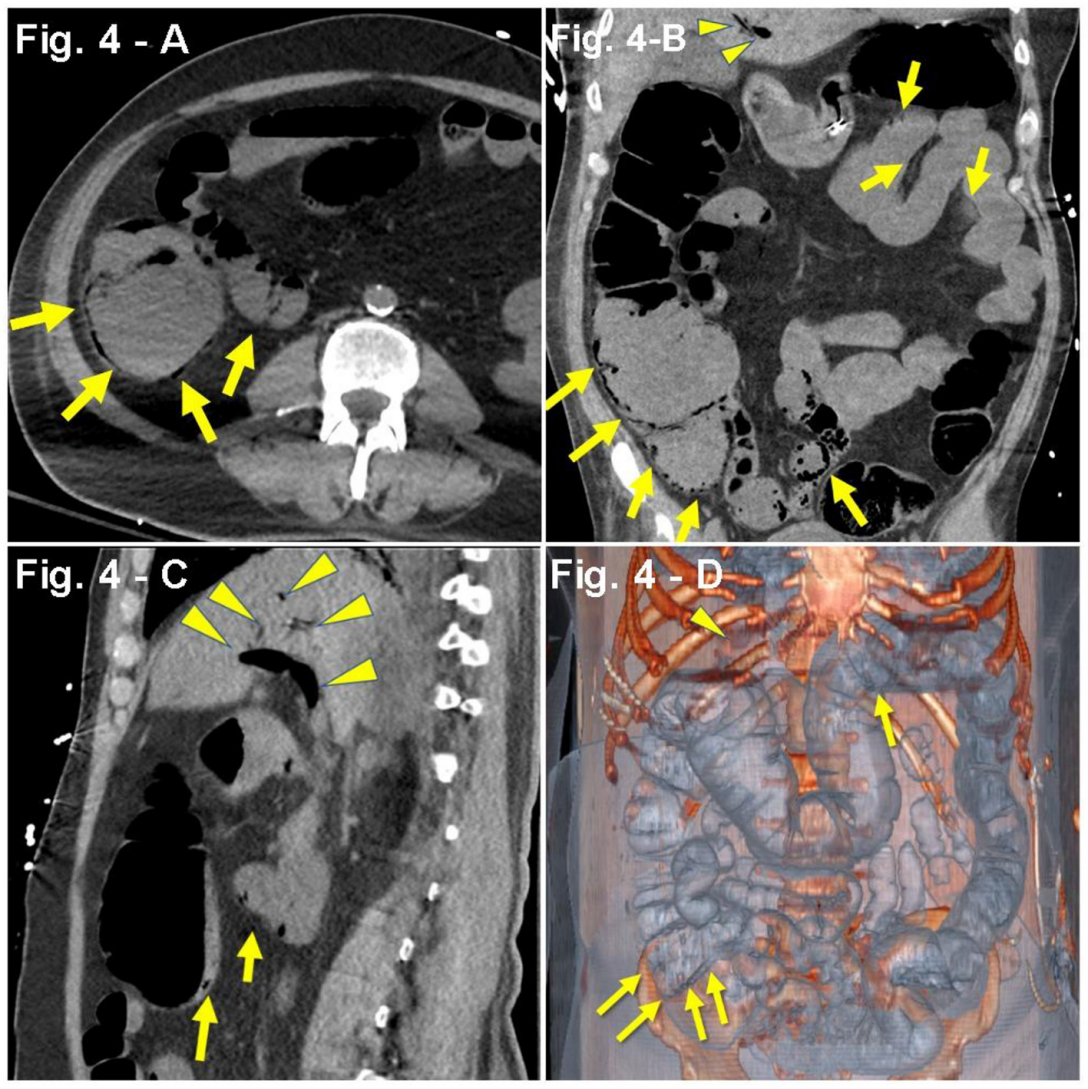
(A–D) Case presentation of a 64-year-old male patient, with altered mental status, acute kidney injury, DM2, stroke, bacterial pneumonia with COVID-19, single-dose TCZ, hypotensive, oliguric, non-contrast abdominal CT only, (A) axial, (B) coronal, (C) sagittal, and (D) 3D reconstruction with pneumatosis of distal transverse colon, cecum, terminal ileum, and mesenteric venous gas adjacent to the terminal ileum (yellow arrows) concerning for bowel ischemia. There are foci of air in mesenteric vessels in the right lower quadrant, with portal venous gas (yellow arrowheads).
